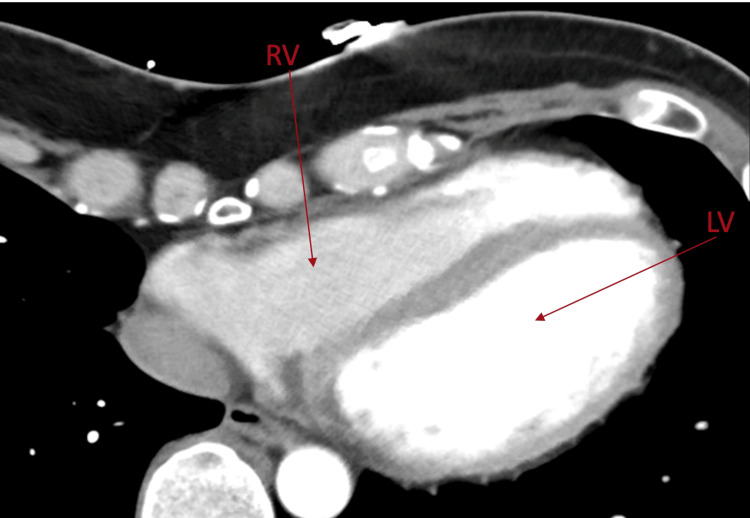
Cardiac CT showing the aneurysmal right ventricular apex, with arrows pointing to the right ventricle (RV) and left ventricle (LV)
